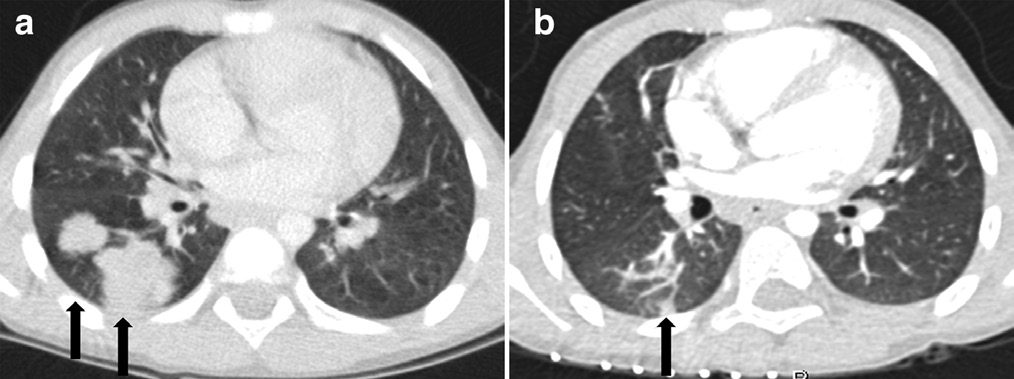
A 7-year-old boy was admitted to the hospital with fever (patient no. two in Table 1). Conventional chest X-ray (not shown) and CT (1A) showed multiple lung lesions (black arrows). On the prebiopsy planning CT (1B), the lesions had either disappeared or considerably decreased in size (black arrow). No biopsy was performed. The patient received antibiotics and completely recovered at 3-month follow-up. The lung lesions were probably attributable to infection.
